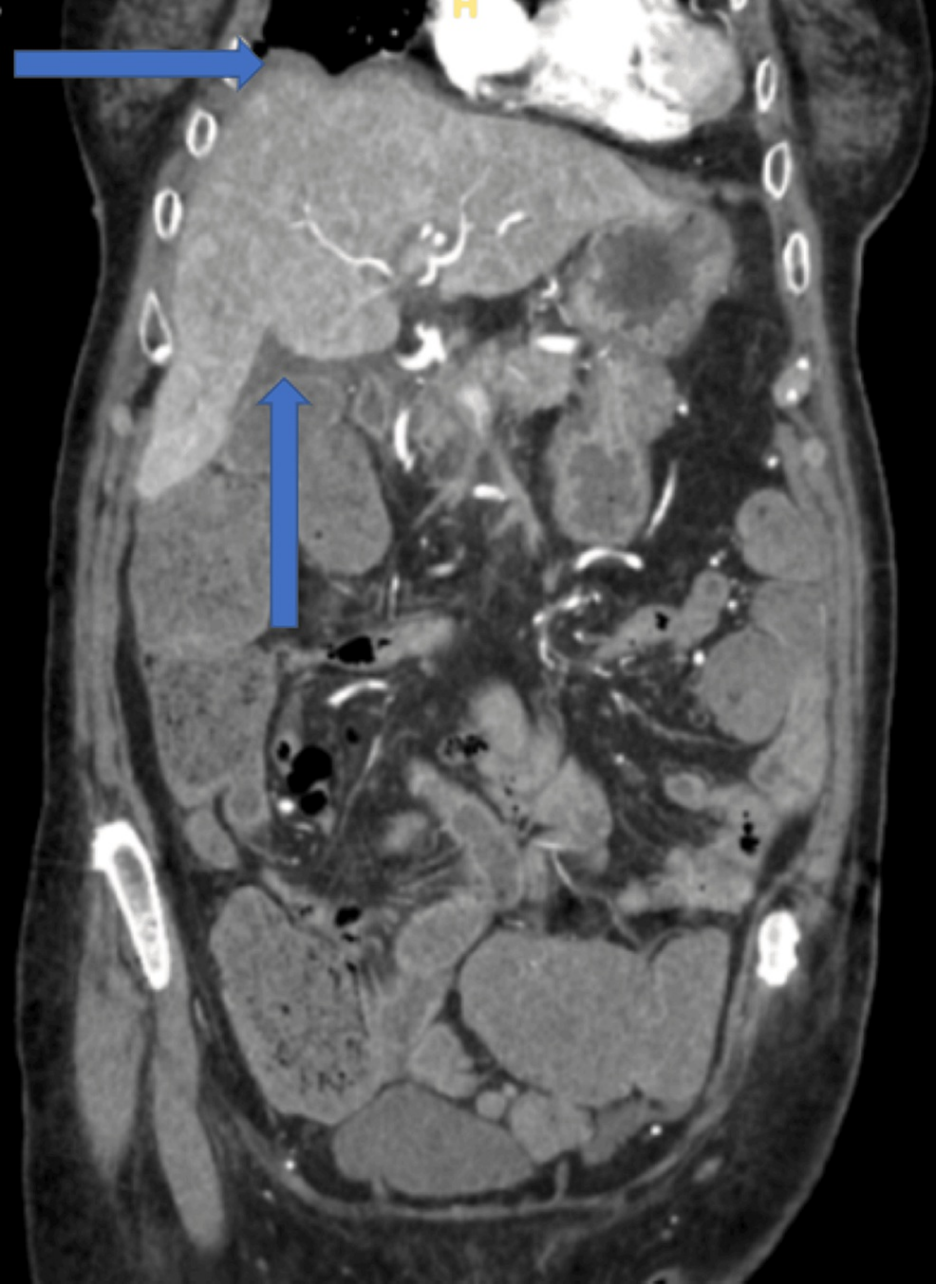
Computed tomography angiography of the abdomen/pelvis (coronal view). Radiologic impression was suggestive of diffuse, multifocal gastroenteric colitis with new ascites and heterogeneous hepatic perfusion.
